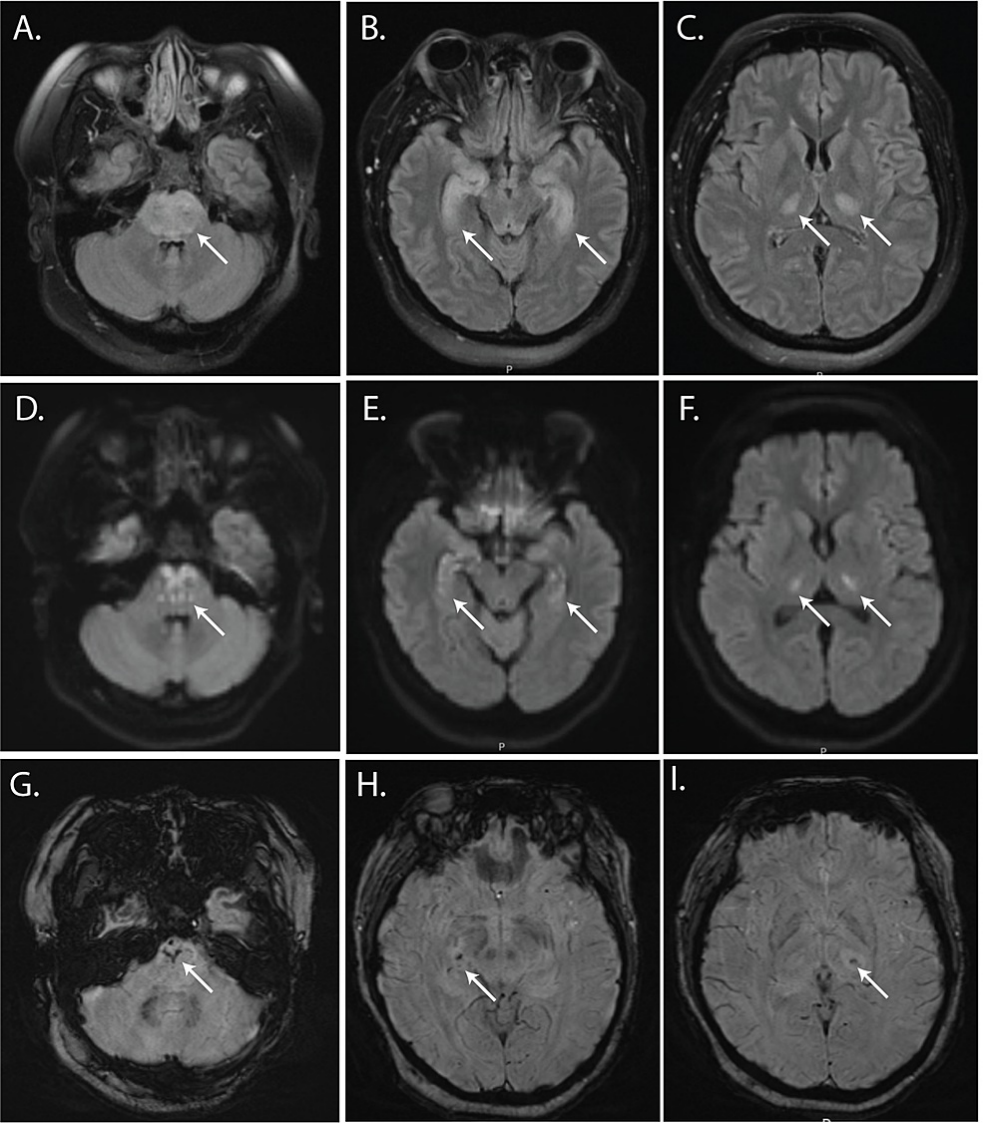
MRI scan on arrival at the hospital. Axial images from MRI obtained on admission showing T2 FLAIR (A-C), diffusion-weighted imaging (DWI) (D-F), and susceptibility weighted imaging (SWI) (G-I) sequences. T2 FLAIR hyperintense lesions contain punctate DWI signal and areas of susceptibility artifact (white arrows).
